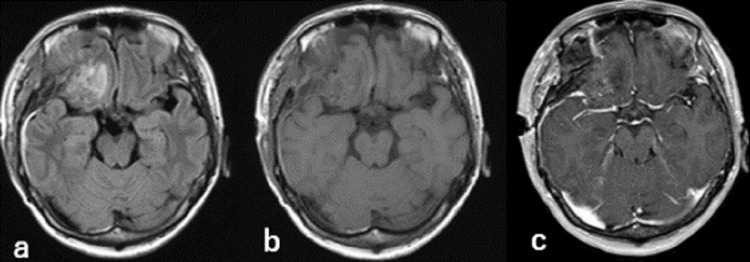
Imaging findings six days after the surgery - (a) FLAIR (b) T1 (c) enhanced MRI FLAIR: fluid-attenuated inversion recovery; MRI: magnetic resonance imaging
